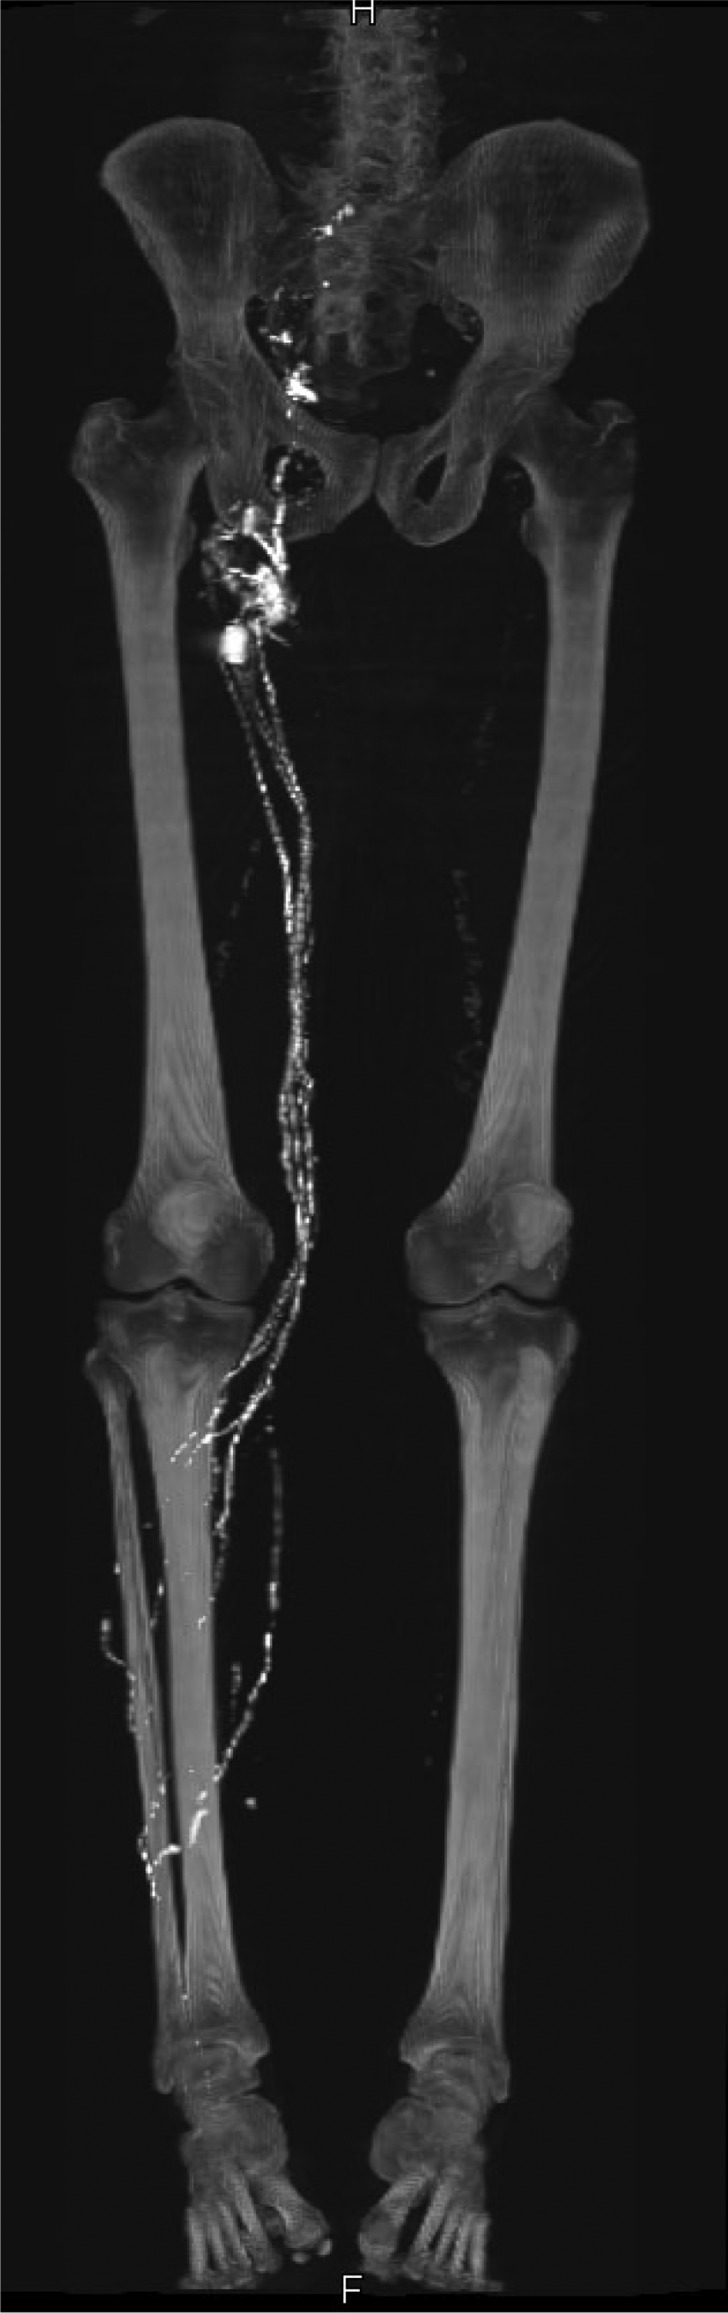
CT imaging after (therapeutic) transpedal lymphangiography for the management of postoperative therapy refractory lymphatic fistula in the right groin.
